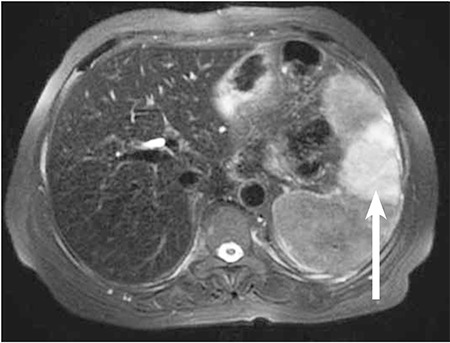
Axial T2-weighted fat-suppressed MRI shows a wedge shaped hyperintense area of infarction in the spleen (arrow), as well as decreased signal intensity of the liver
